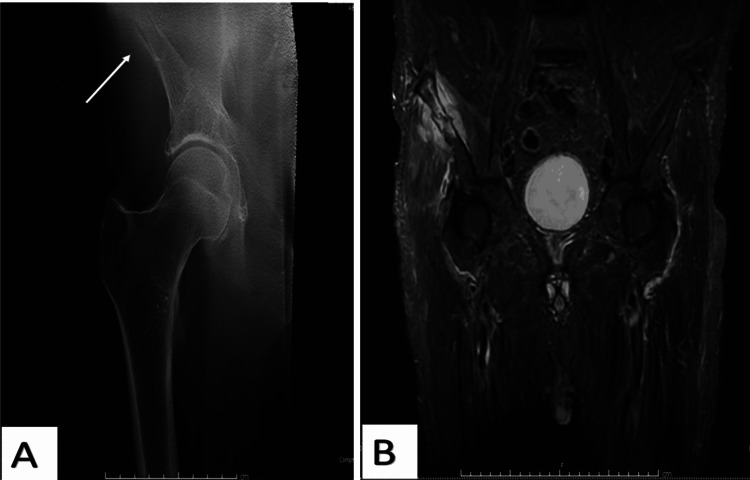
An 80-year-old male patient had a mechanical fall and presented with right hip pain. The tomogram showed an acute fracture affecting the right iliac wing (A, arrow). MRI showed a fracture affecting the right iliac wing with surrounding hematoma. An acute muscular strain injury is also noted within the gluteal muscles on the right. There are osteoarthritic changes in both hips (B).
